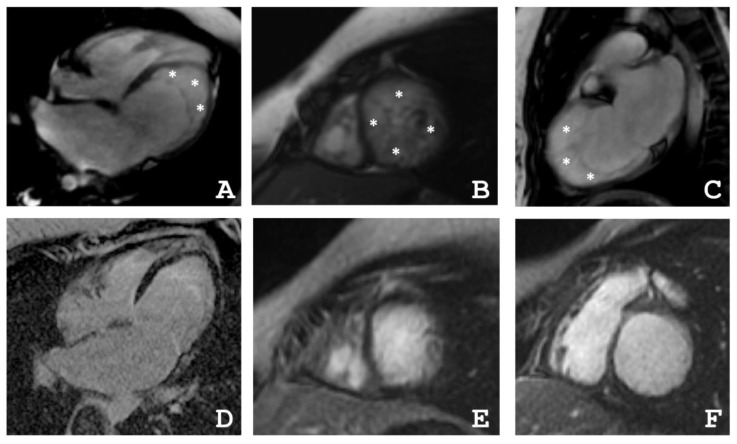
(A–C) Proband’s daughter (IV-3) cardiac magnetic resonance imaging in movie mode, SSFP sequence: (A) long axis 4–chamber projection, (B) short axis at the level of the apical segments, (C) long axis 2–chamber projection, * indicates the layer of non-compact myocardium, (D,F) delayed contrast. Inversion recovery sequence with suppression of the signal from the myocardium: (D) long axis 4–chamber projection, (E) short axis at the level of apical segments, (F) short axis at the level of basal segments. There were no areas of contrast, which indicates the absence of areas of intramyocardial fibrosis, scarring, and inflammatory myocardial damage.
